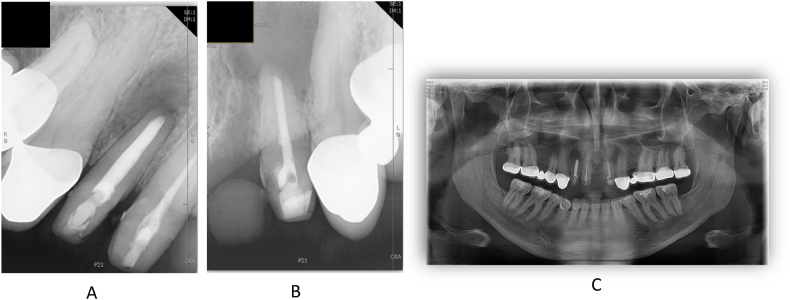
Postoperativ periapical x-ray about the 12 tooth (A), about the 22 tooth (B) and the orthopantomogram (C).
